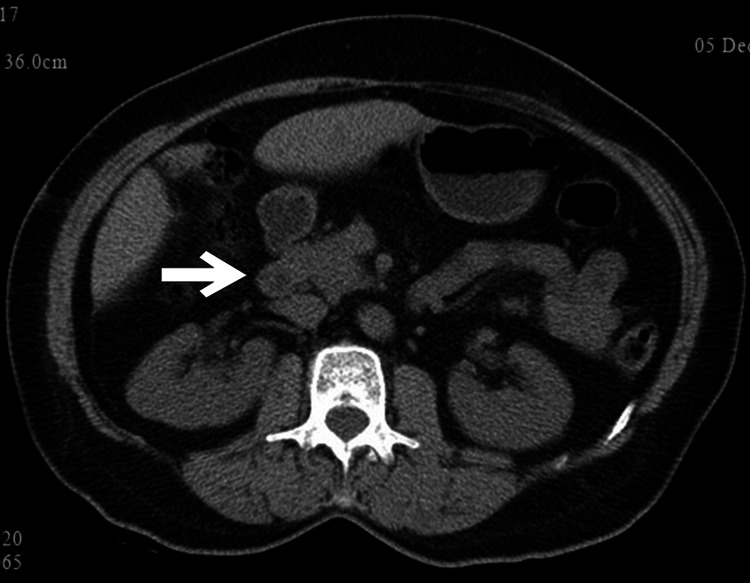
Plain CT image showing a homogeneous lesion in the pancreatic head.
